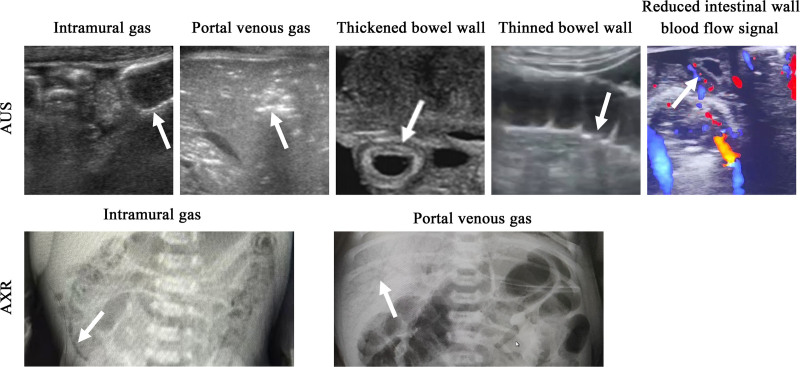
Representative AUS and AXR images of NEC. The white arrow indicates the target feature. AUS = abdominal ultrasound, AXR = abdominal X-ray, NEC = necrotizing enterocolitis.
